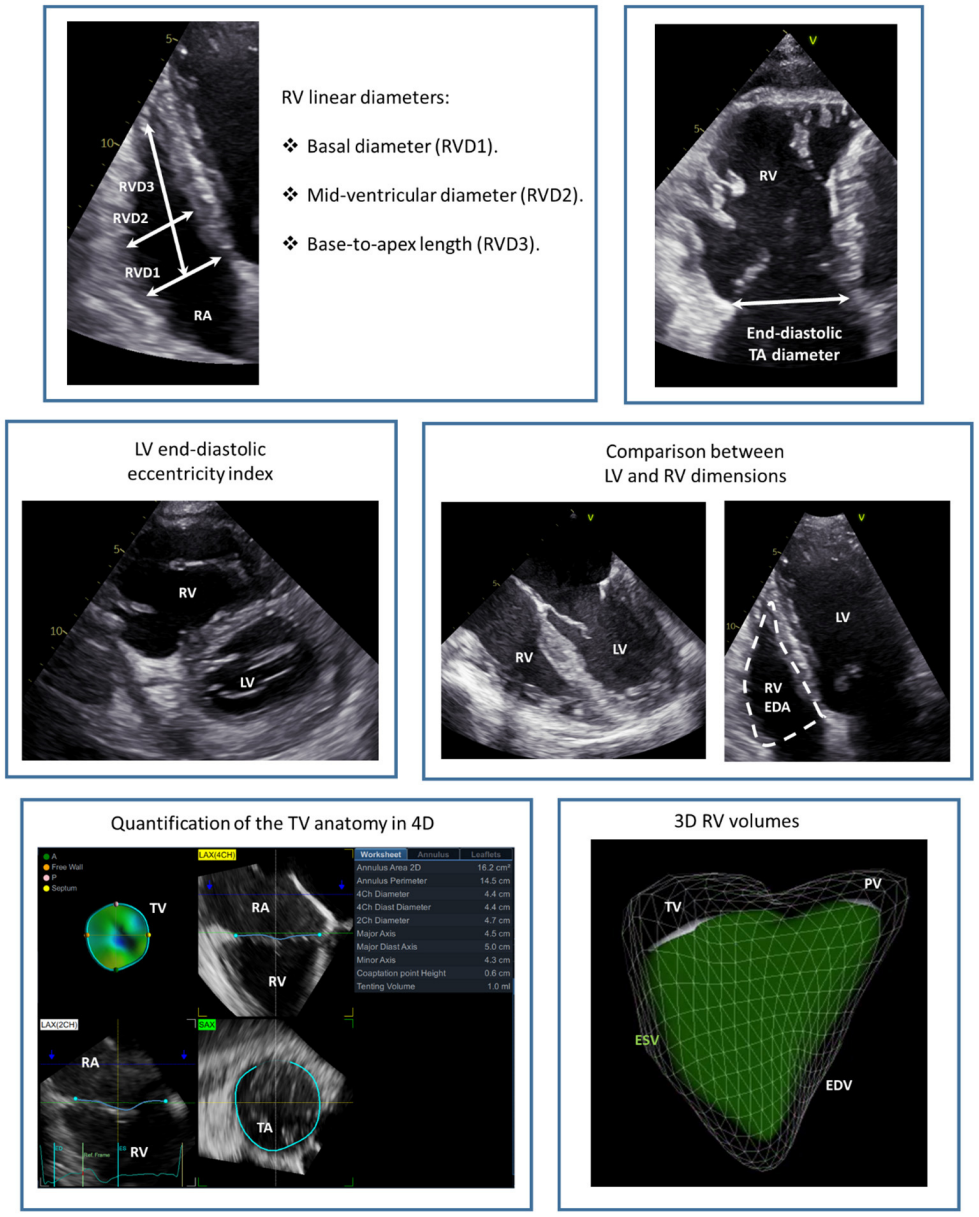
Echocardiographic assessment of the tricuspid annulus and right ventricular dimensions. The figure presents a summary of the main echocardiographic measurements of RV and TA dimensions. EDA, end-diastolic area; EDV, end-diastolic volume; ESV, end-systolic volume; LV, left ventricle; PV, pulmonary valve; RA, right atrium; RV, right ventricle; RVD, right ventricular diameter; TA, tricuspid annulus; TV, tricuspid valve.
